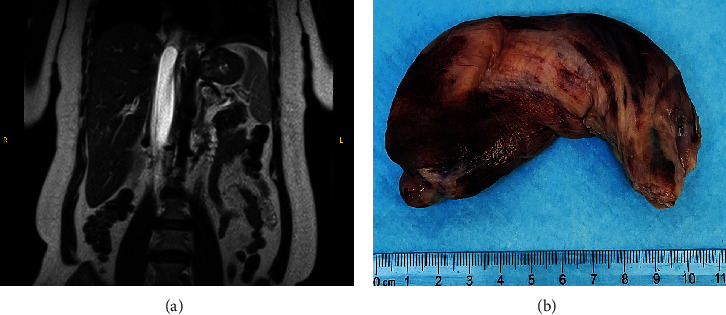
Radiology and gross tumor examination. (a) T2 magnetic resonance imaging (MRI) shows extensive venous thrombus extending from the right internal iliac vein to the right atrium, representing tumoral thrombosis or an intravenous mass inferiorly with transition into bland thrombus at the level of the intrahepatic inferior vena cava (IVC). (b) A gross examination of the mass measures around 10 cm.
